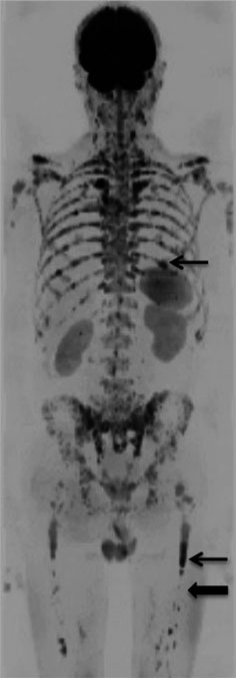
Whole body diffusion weighted magnetic resonance imaging (WB DW‐MRI) provides excellent contrast between normal bone marrow and focal lesions. b900 WB DW‐MRI Maximum Intensity Projection image in a 55‐year‐old male with multiple myeloma demonstrates multiple focal lesions that appear low signal on the inverted grey scale image (examples indicated by arrows) with excellent contrast against normal marrow, which does not return signal (block arrow).
